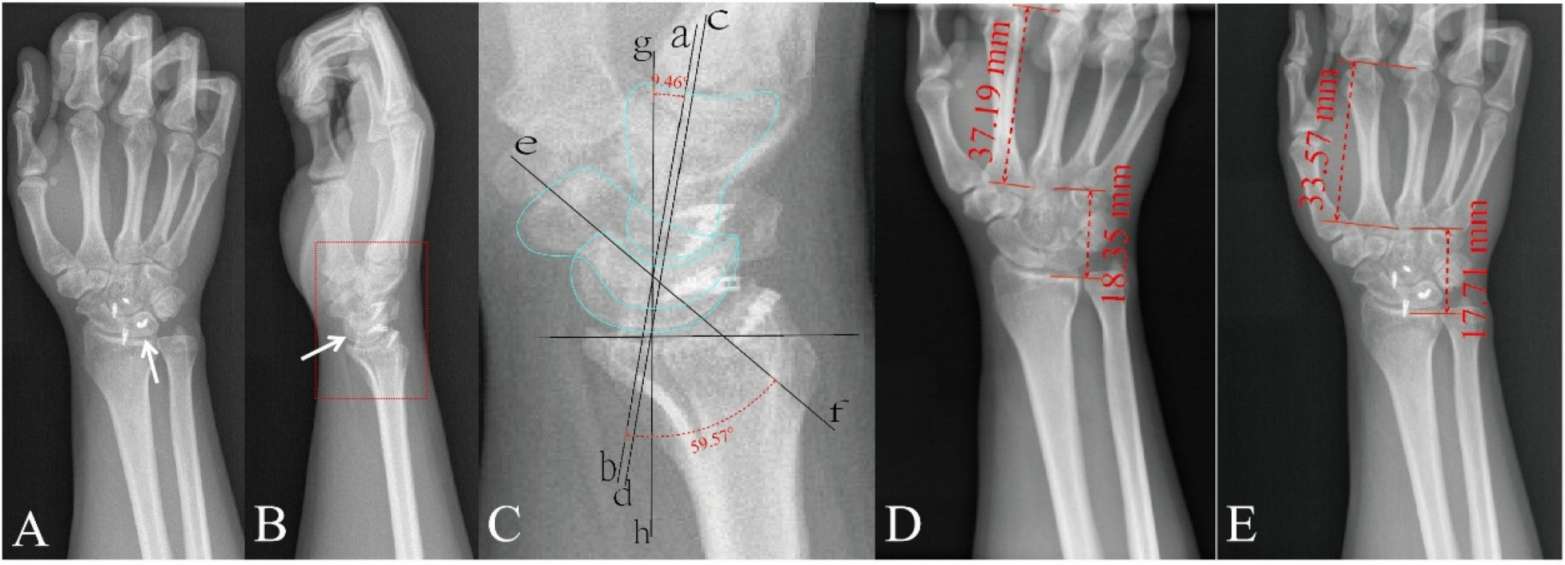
Postoperative one-year follow-up radiographs: posteroanterior radiograph (A) and lateral radiograph (B, C) is a magnified view of the red box in (B), showing detailed anatomy. Preoperative posteroanterior radiograph (D) for comparison. Postoperative posteroanterior radiograph (E) White arrows indicate lunate reduction. The measured distance marked in red represents the carpal height and the third metacarpal length. Carpal Height Ratio = Carpal Height/Third Metacarpal Length. Radiolunate angle: 9.46° (angle formed by lunate axis ab and radius axis gh). Capitolunate angle: 0° (angle formed by capitate axis cd and lunate axis ab). Scapholunate angle: 59.57° (angle formed by scaphoid axis ef and lunate axis ab).
